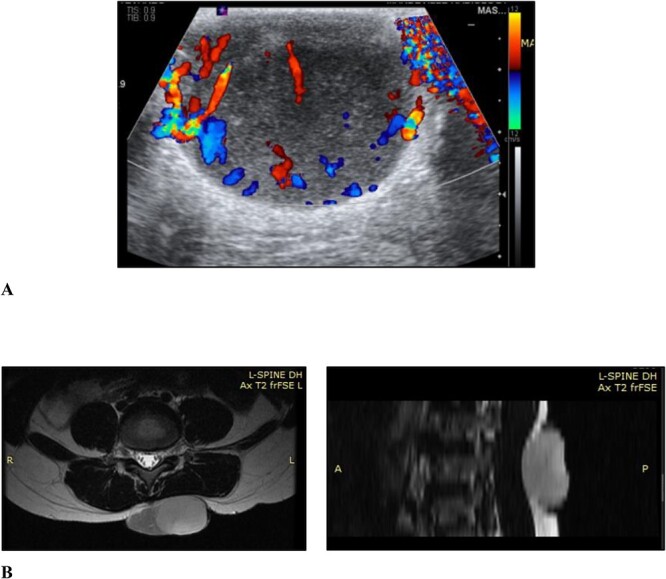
(A) Ultrasound scan of the lesion to the lower back with doppler showing vascular flow; (B) MRI scan of the lesion to the back showing it extending to the level of the erector spinae fascia but not crossing it and no tract to the spinal canal, in the axial and sagittal planes, respectively.
